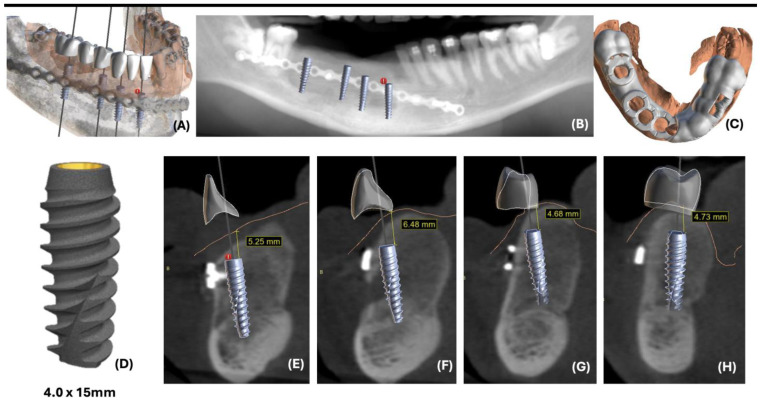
(A) Digital planning showing the implants oriented prosthetically; (B) Radiographic view of the planned implants; (C) surgical guide; (D) dental implant; (E–H) sagittal view of the implants placed in positions 41, 43, 44, and 46 respectively.
